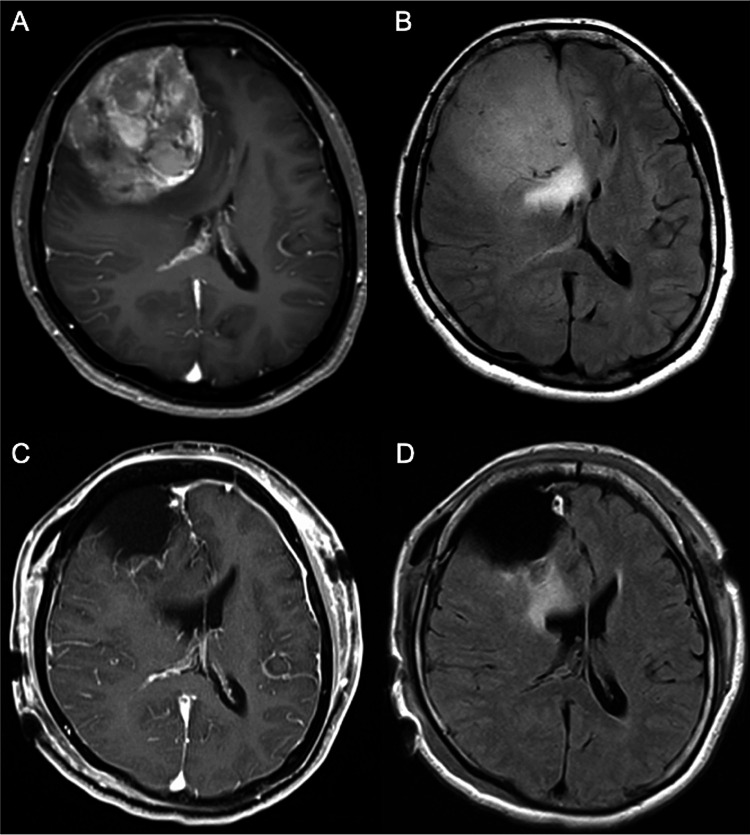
Pre- and postoperative magnetic resonance images of Case 2. (A) Preoperative Gd enhanced axial T1WI. (B) Preoperative axial FLAIR image. (C) Postoperative Gd enhanced axial T1WI. (D) Postoperative axial FLAIR image. Gd: gadolinium; T1WI: T1-weighted image; FLAIR: fluid-attenuated inversion recovery
